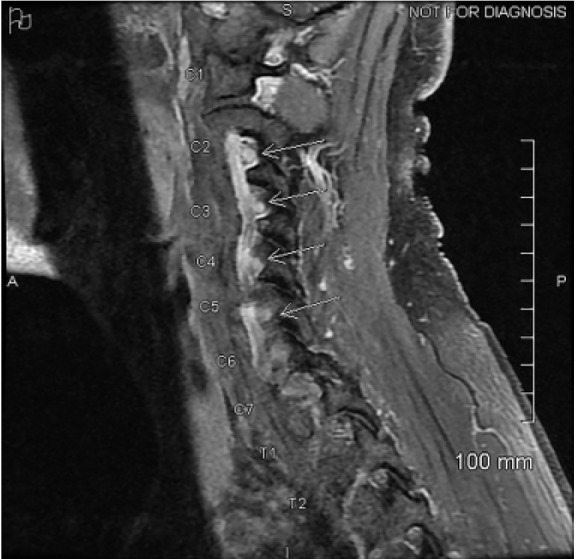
Magnetic resonance imaging of the cervical spine with and without contrast revealed enhancement of the cervical spine nerve roots (arrows), which may be seen in acute inflammatory demyelinating polyneuropathy.
